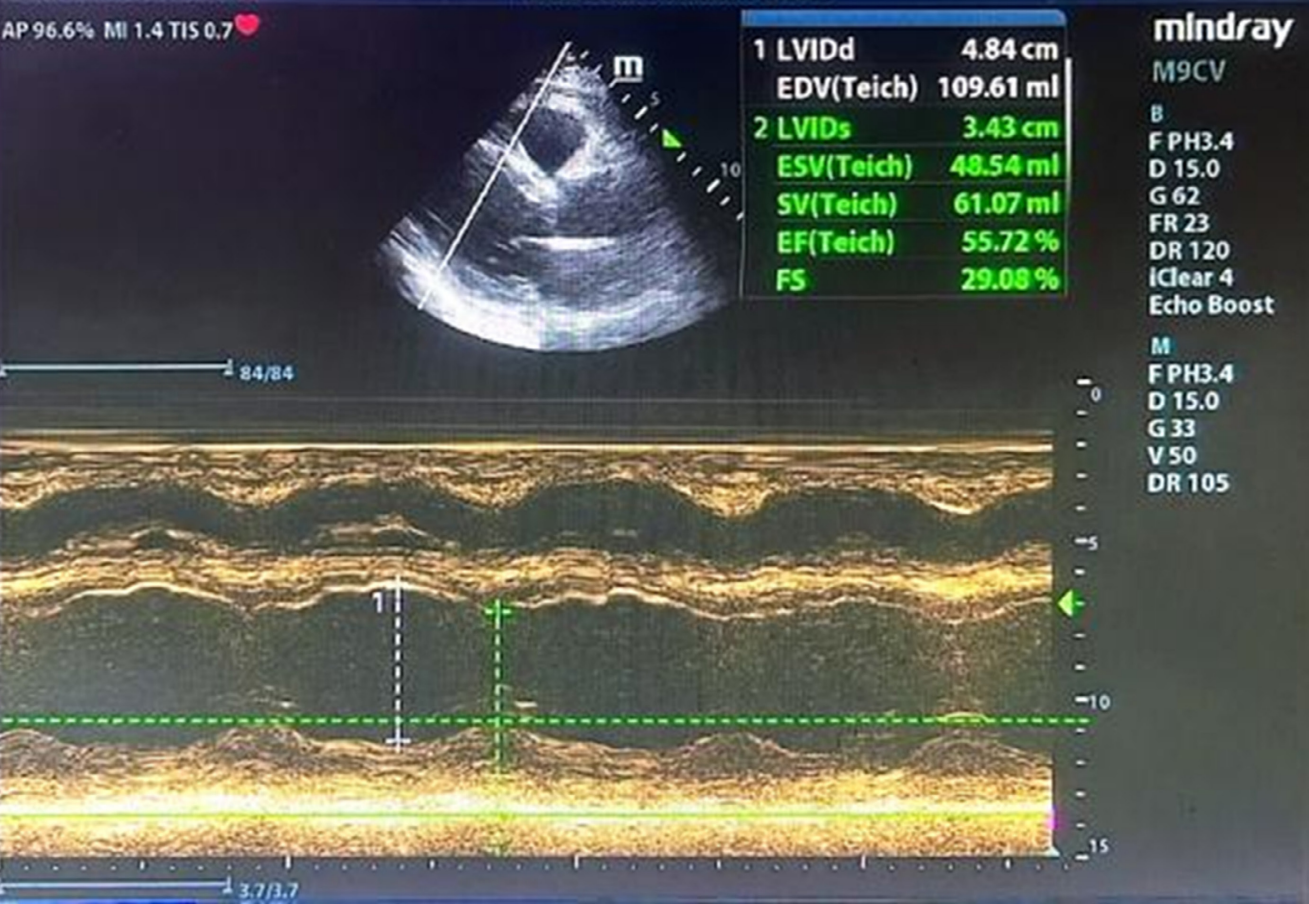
Echocardiography after percutaneous coronary intervention. Left Ventricular Internal Diameter in Diastole (LVIDd): 4.84 cm, End‐Diastolic Volume (EDV): 109.61 mL, Left Ventricular Internal Diameter in Systole (LVIDs): 3.43 cm, End‐Systolic Volume (ESV): 48.54 mL, Left ventricular stroke volume (SV): 61.07 mL, Left ventricular ejection fraction (LVEF): 55.72%, Fractional Shortening (FS): 29.08%.
